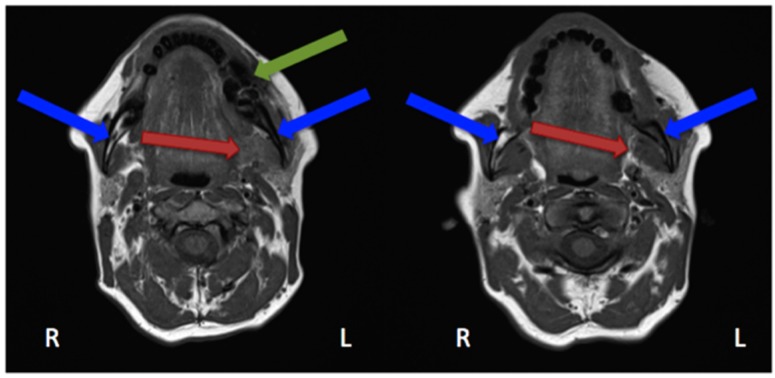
Magnetic resonance imaging (Siemens, Avanto, 1.5T, Sequence: T1 tse tra) Patient: 48 years old, female, metastatic breast cancer, zoledronic acid for 2 years (panoramic radiograph Figure 1). Green arrow showing the MRONJ necrosis, red arrows showing the oedema, blue arrows showing the differences between the right side: normal fatty bone marrow and left side: signal loss, due to loss of fat. Pair of screenshots. AAMOS staging: stage 2.
